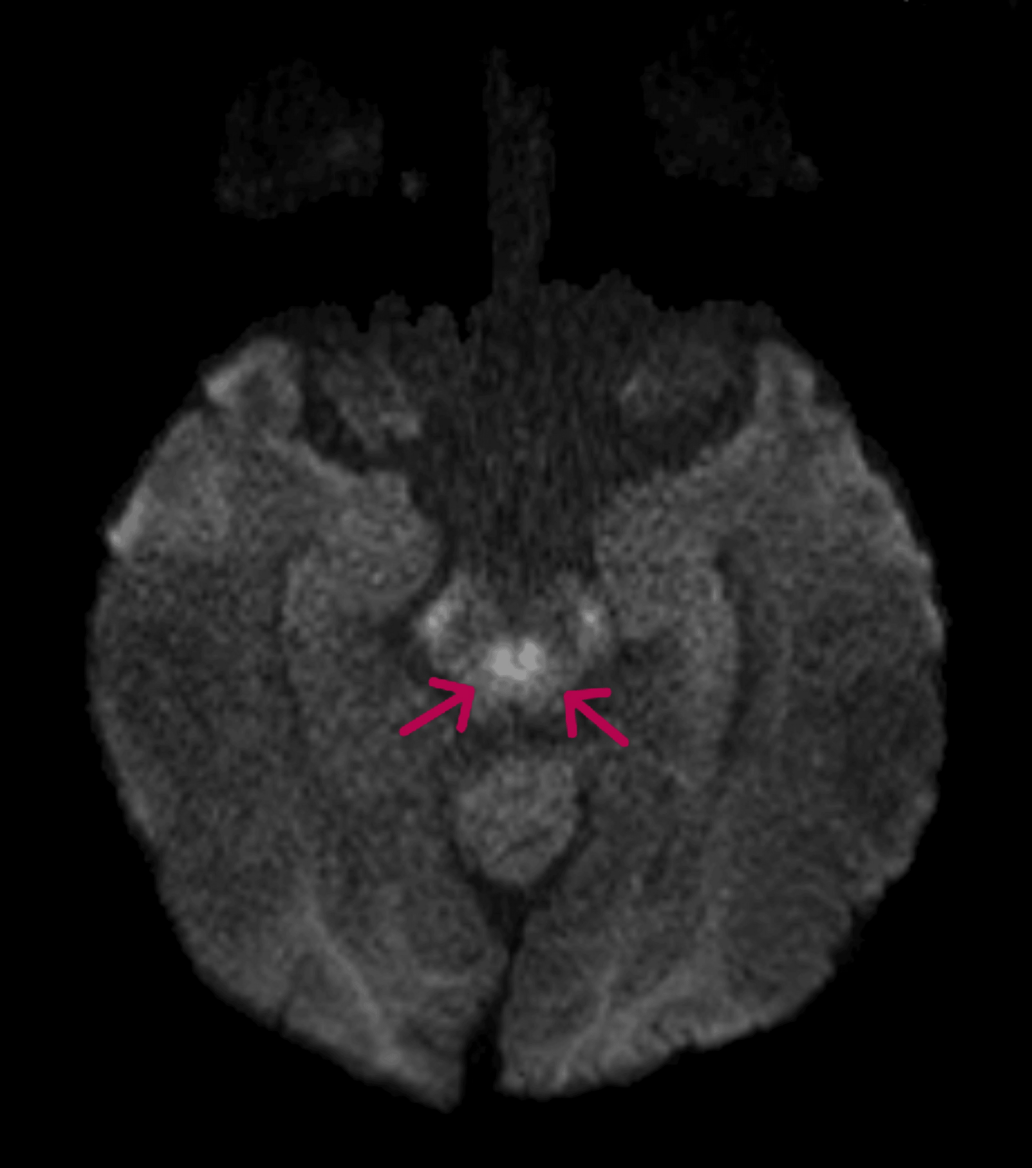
MRI brain (DWI sequence) shows diffusion hyperintensity in rostral midbrain (arrows). DWI: Diffusion-weighted imaging
